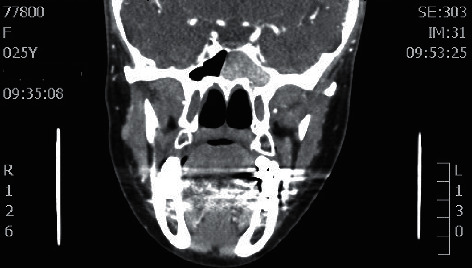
Coronal CT sinuses showing left sphenoid sinus fungal ball.
